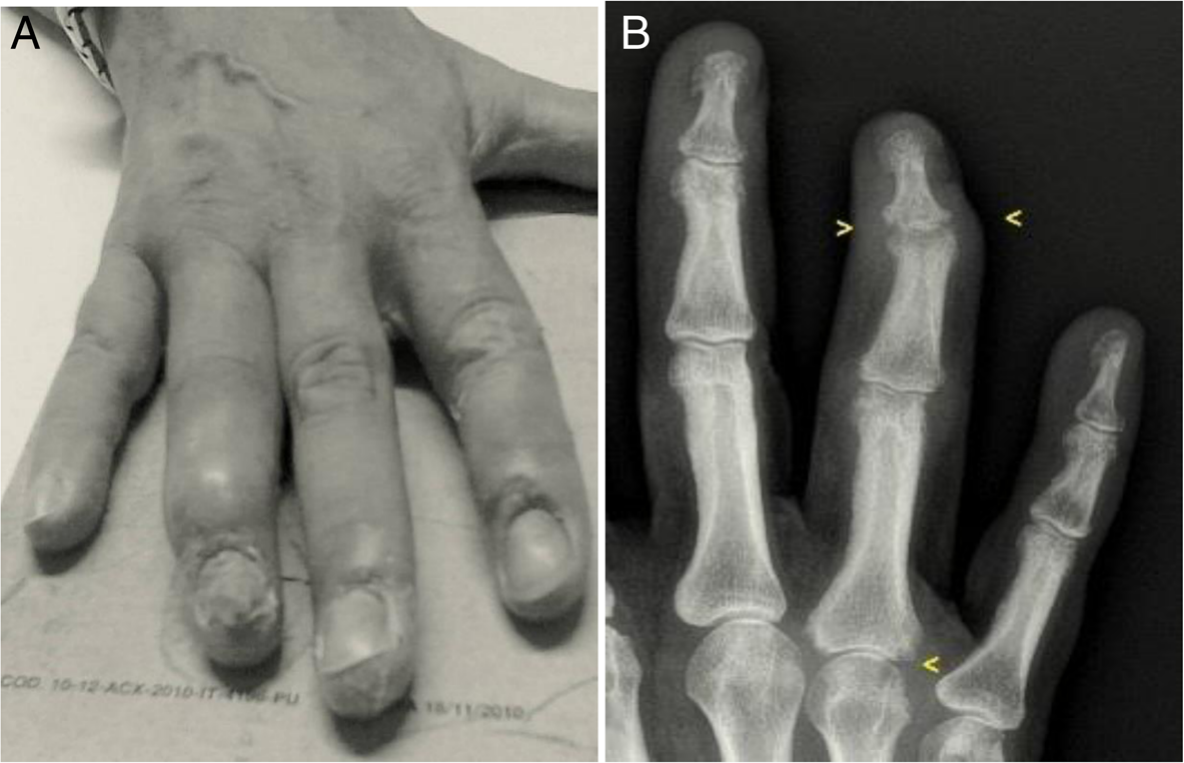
Right hand: arthritis of the fourth distal interphalangeal joint with severe nail psoriasis (a). Radiograph of the right hand: erosions in the fourth metacarpophalangeal and distal interphalangeal joints (b)
